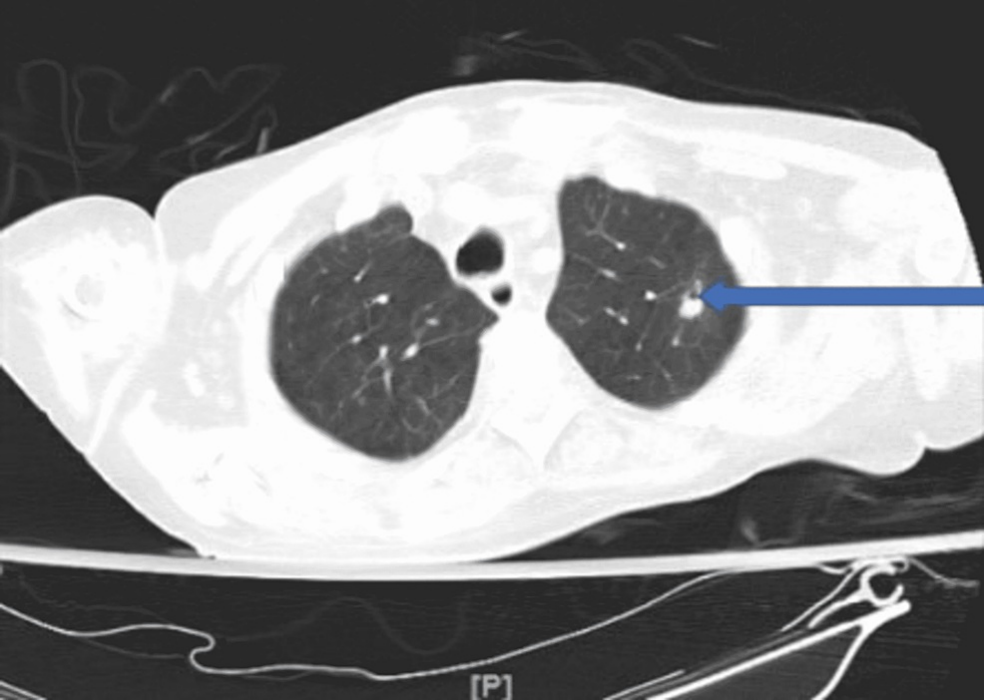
CT showing cavitary lesion in the apical posterior segment of the left upper lobe (blue arrow) taken during patient admission.
